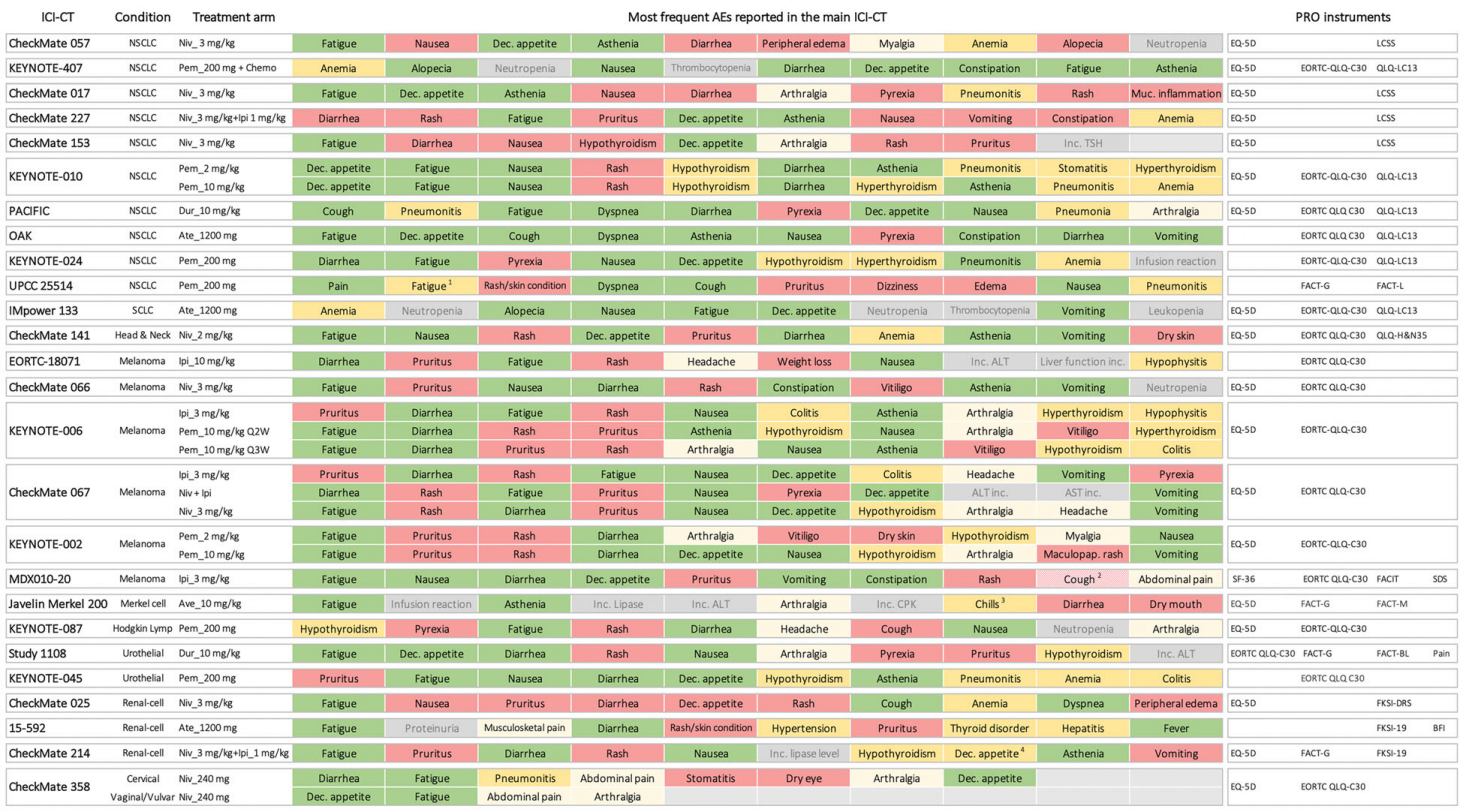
Comparison of PRO instruments’ symptom-related content and AEs reported in ICI-CTs with published PRO results (*n* = 26). The most frequently reported AEs (any grade) are shown for the 26 ICI-CTs identified. AE frequency (most common to least common) is depicted from left to right respectively. Symptom-related content from each PRO instrument was compared to the AEs in the corresponding trial arm. The PRO instruments used in each ICI-CT is shown. For a detailed PRO instrument content, see Table S8. Green: AEs covered; Yellow: AEs partially covered; Light yellow: AEs partially covered related to specific types of pain; Red: AEs not covered. Laboratory tests results (grey) were not included in calculations. “Asthenia” was considered covered by “fatigue” following the National Cancer Institute toxicity grading scale version 3 that included asthenia, lethargy, and malaise under the umbrella of “fatigue”. (1) “Fatigue” was considered partially covered because the term included in the FACT-G was “lack of energy”; (2) While the study protocol announced the use of SF-36, EORTC QLQ-C30, FACIT-Fatigue, and SDS questionnaires, only results from the EORTC QLQ-C30 were reported in the full-text publication. “Cough” would be covered by the SDS instrument; (3) “Chills” was partially covered as related to “fever”, included in the FACT-G; (4) “Dec. appetite” was considered as partially covered by the term “good appetite” in the FKSI-19. Ipi, Ipilimumab; Niv, Nivolumab; Pem, Pembrolizumab; Ate, Atezolizumab; Dur, Durvalumab; Inc., increased; Dec, decreased
